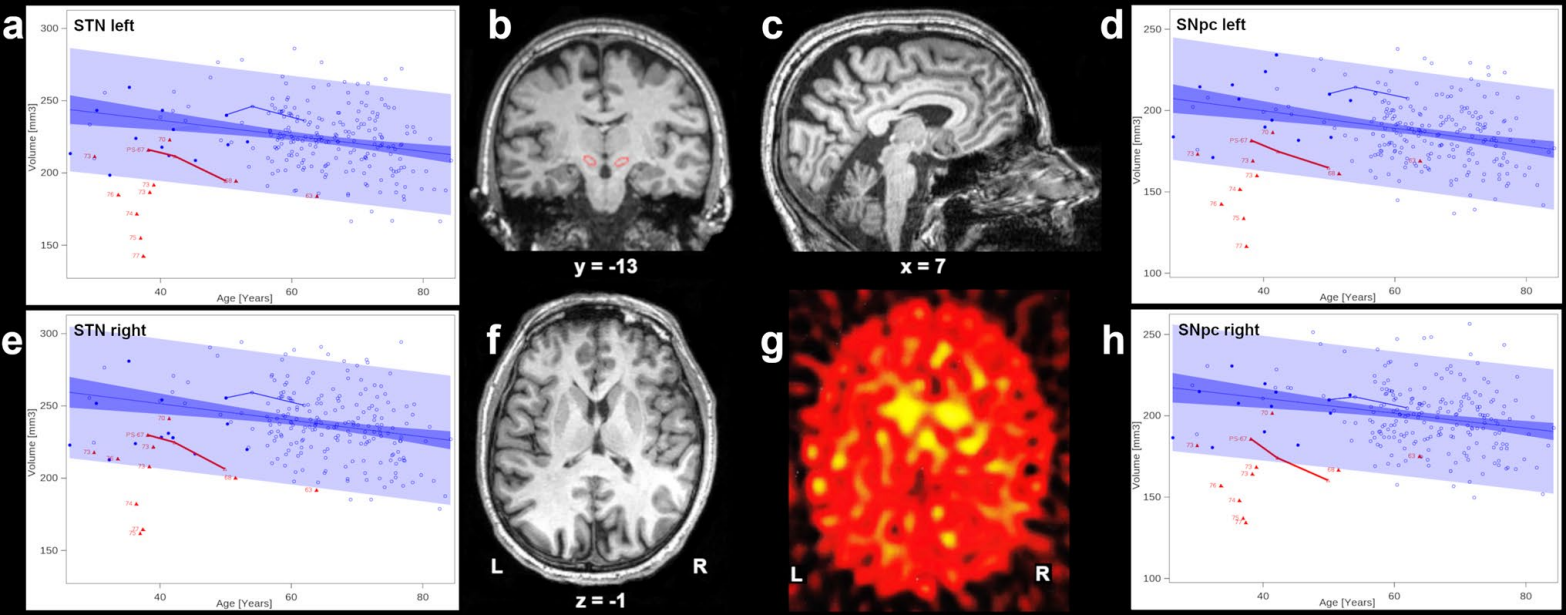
b, c, f 3 T MRI data of the SCA3 patient with parkinsonian symptoms at age 50, outlining in red the anatomical position of the right and left STN (b) demonstrating moderate cerebellar atrophy (c) and normal-appearing basal ganglia (f). g Dopamine transporter SPECT revealed bilateral loss of striatal uptake. a, e, d, h MRI-based volumes of the STN (a, e) and SNpc (d, h) in SCA3 patients (red, numbers indicate the individual repeat length of the expanded allele), age- and sex-matched healthy controls and elderly healthy participants of the 1000BRAINS study (blue). Filled circles and triangles refer to MRI data obtained at 1.5 T and non-filled circles and triangles to data obtained at 3 T. The blue area surrounding the age-dependent regression line represents the confidence interval of the age-dependent mean values of all control subjects. Longitudinal STN volume data of the single SCA3 patient with parkinsonian symptoms (red; data obtained 12 years and 8 years prior to DBS and shortly before DBS) and one healthy control at three different time points with similar intervals in between are connected by lines.
